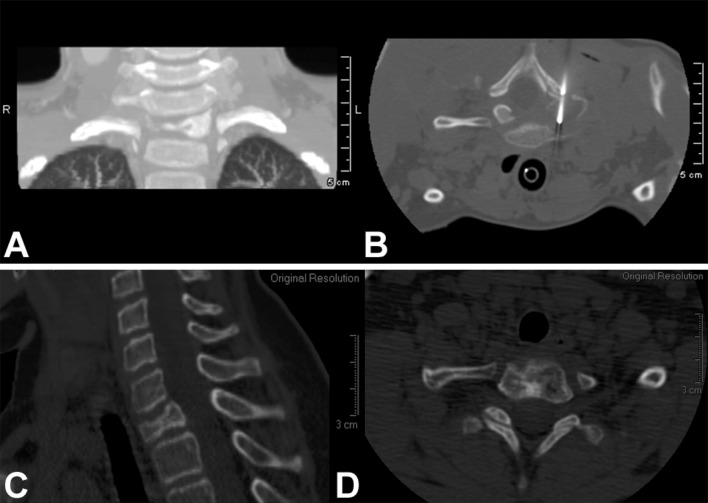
a Coronal CT scan of the cervical spine of a 5-year-old boy with a painful osteolytic lesion of the C7 vertebral body. b CT-guided frozen section biopsy showed eosinophilic granuloma; intralesional methylprednisolone injection was performed. c, d Sagittal (c)and axial (d) CT scans showing complete reconstitution of the lesion 5 years after diagnosis and treatment
